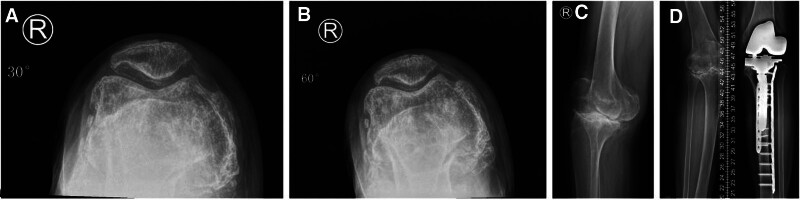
The X-ray was taken 3 months after the surgery. ((A and B) Left knee flexion = 30° and 60°. (C) Side position of the right knee joint. (D) The X-ray of bilateral knee joint.).
